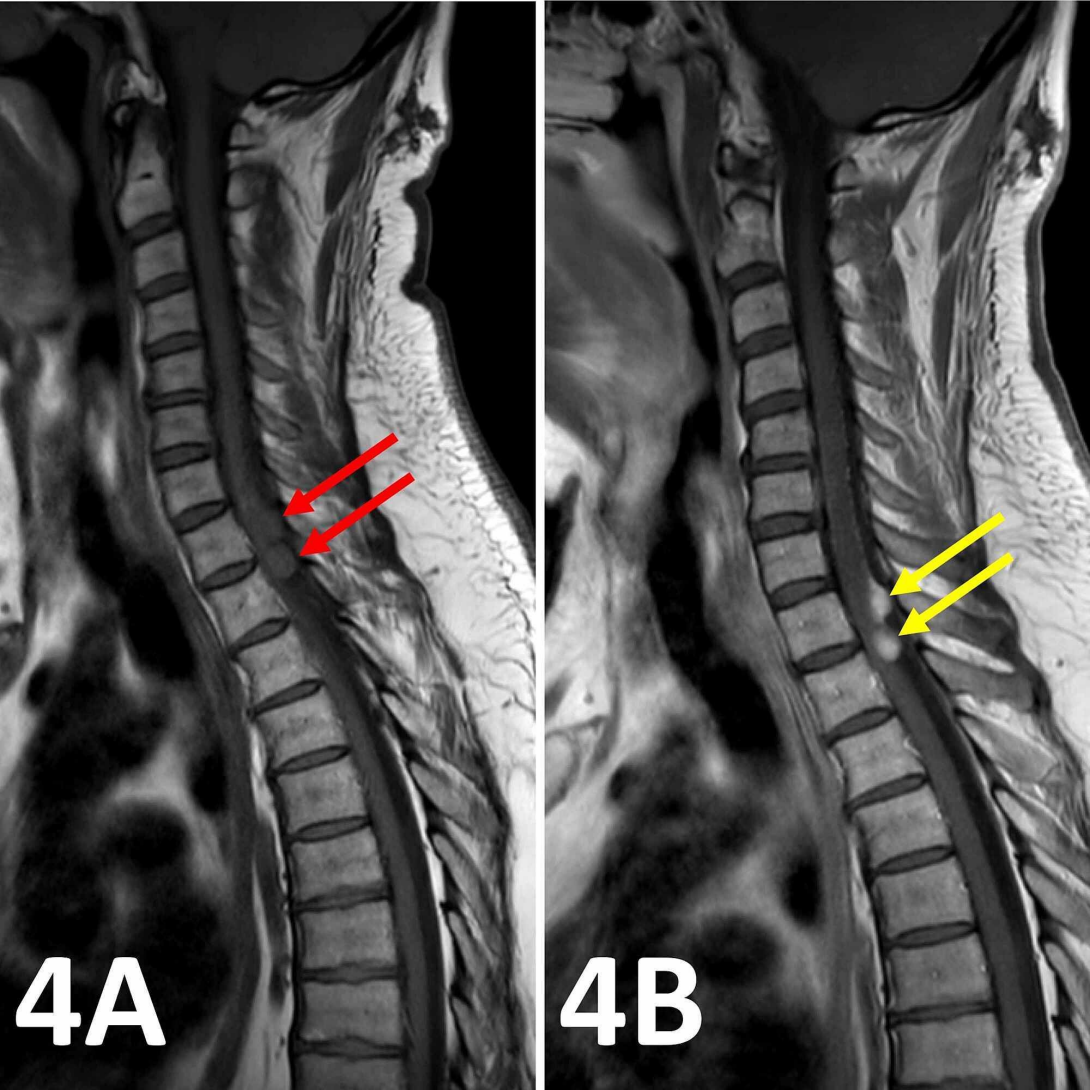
Sagittal T1 pre- and post-contrast spinal MRI images. (A) Sagittal T1 pre-contrast spinal MRI image showing two isointense intradural lesions at the T1/T2 vertebral level (red arrows). (B) Post-gadolinium administration, the two lesions enhance homogenously highlighting their intramedullary and extradural elements (yellow arrows).
